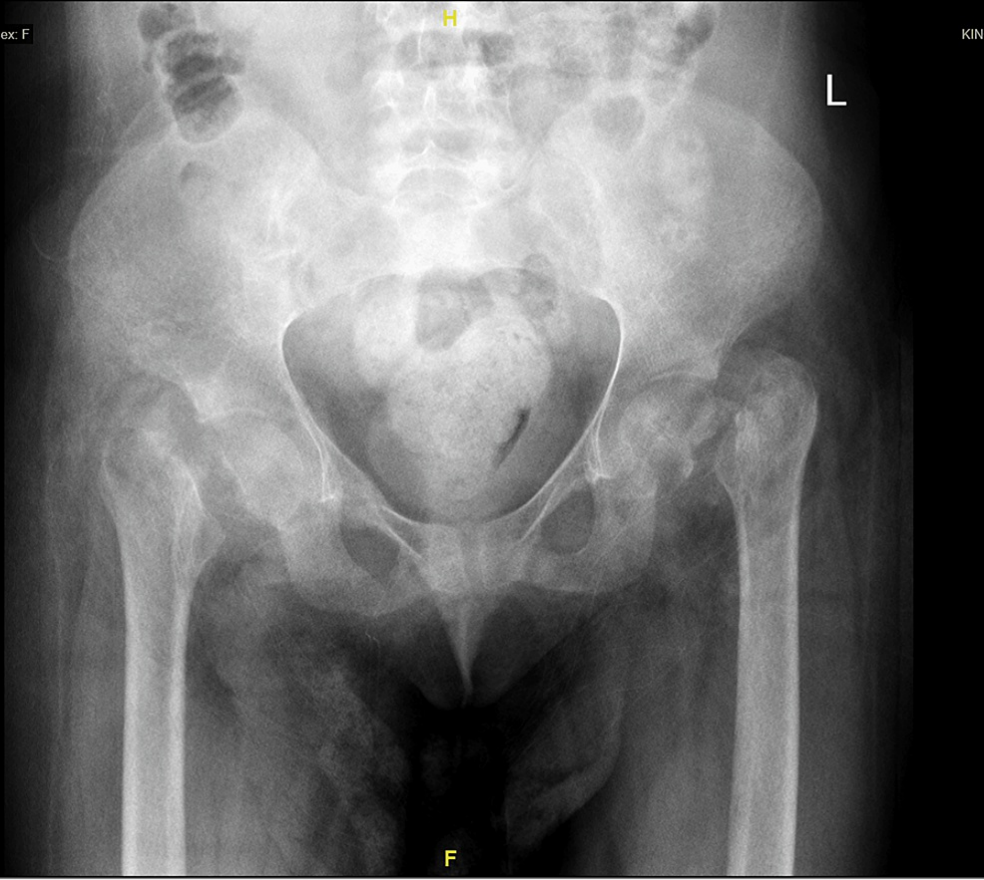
Radiograph of the pelvis on presentation to the emergency room two months after the injury showing bilateral femoral neck fractures.
